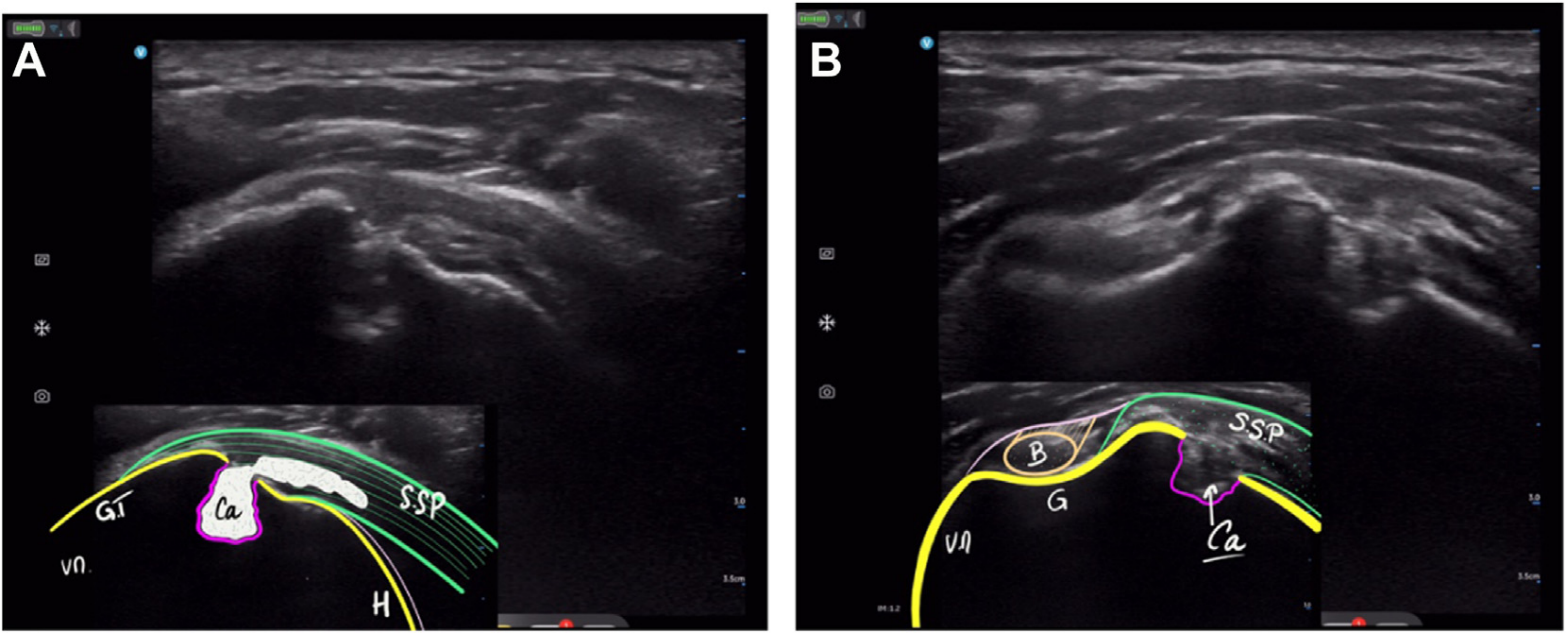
Ultrasound images performed by the surgeon during a consultation showing a calcium deposit in the supraspinatus tendon with intraosseous extension. (A) Longitudinal view. (B) Transverse view. *GT*, great tubercle; *H*, head; *Ca*, calcium deposits; *SSP*, supraspinatus tendon; *B*, biceps; *G*, gutter.
